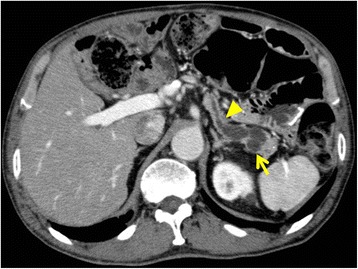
Computed tomography. A caliber change in the MPD at the pancreatic body was detected (*arrow head*). The cyst was located in the pancreatic tail (*arrow*)
